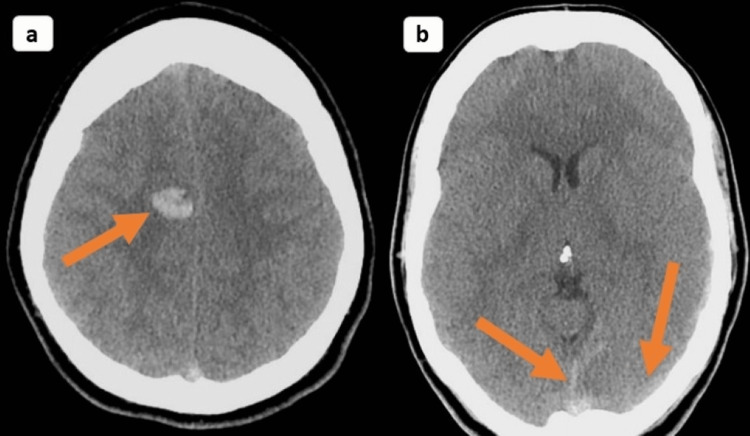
CT revealing a hyperintense signal intensity alteration in the right frontal lobe suggestive of a hemorrhagic infarct and multiple filling defects in the superior sagittal sinus and the transverse sinus suggestive of cerebral venous sinus thrombosis
